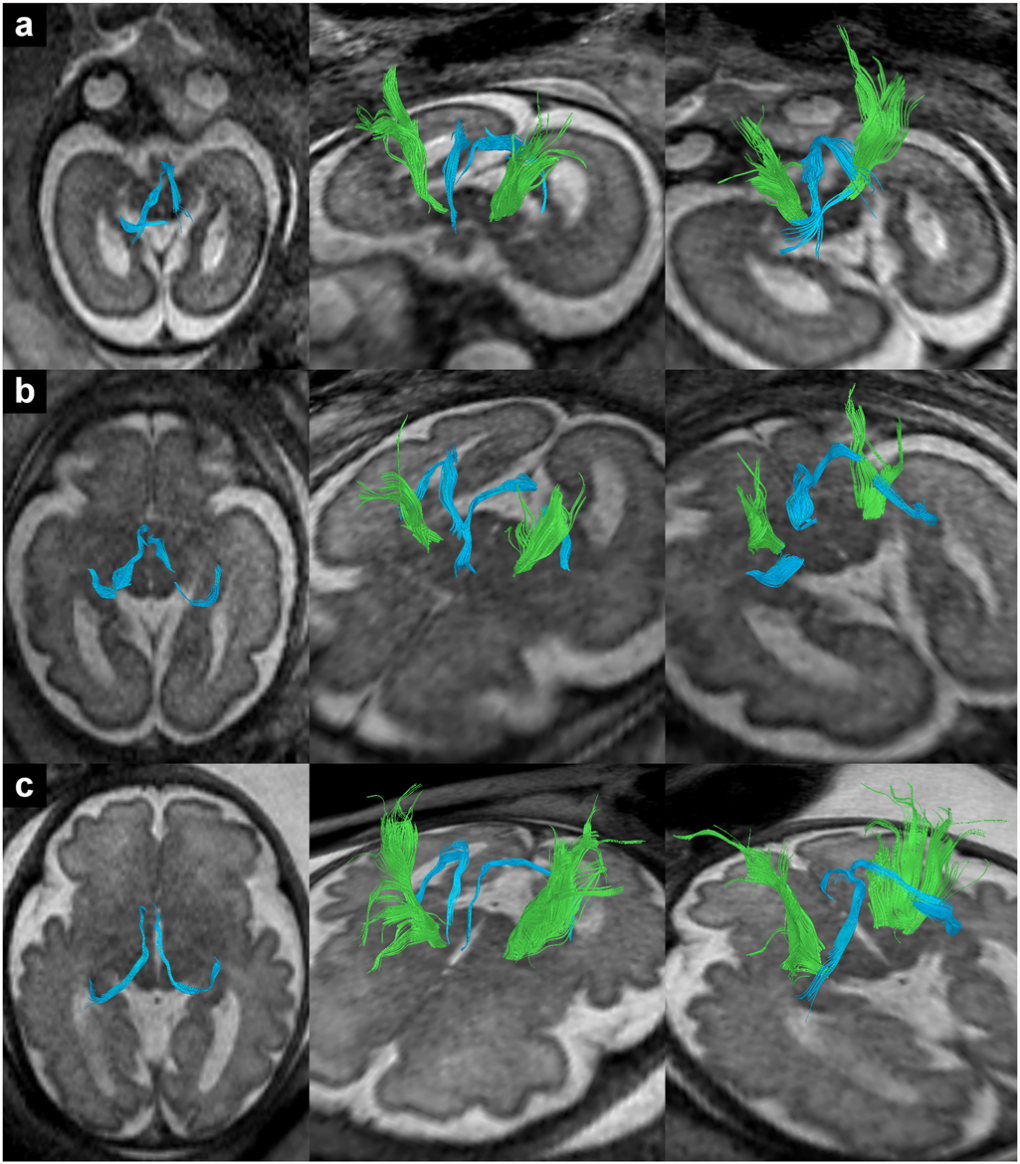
In utero tractography of the fornix (light blue) in subjects 14 (a), 17 (b) and 23 (c) (27, 30 and 33 GW respectively) and its relation to corticospinal/ corticopontine fibers (green). Tractography of corticospinal/ corticopontine fibers was performed according to the methods described in Kasprian et al. (2008) [26]. Fiber tracts are projected on axial T2-weighted images.
